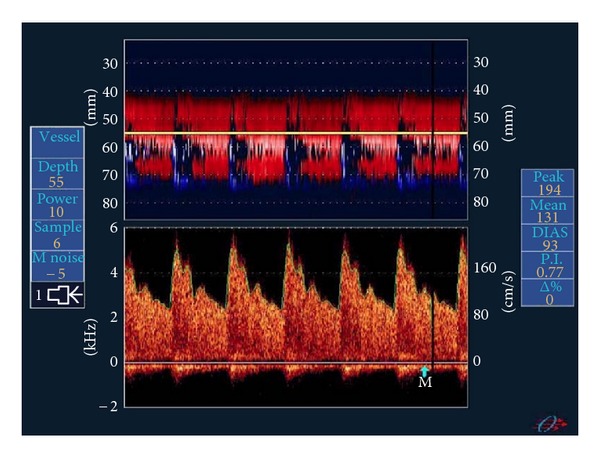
TCD. Doppler velocities demonstrating moderate MCA vasospasm using M mode (above) and pulse-wave Dopper (below). This figure is reproduced from [7].
